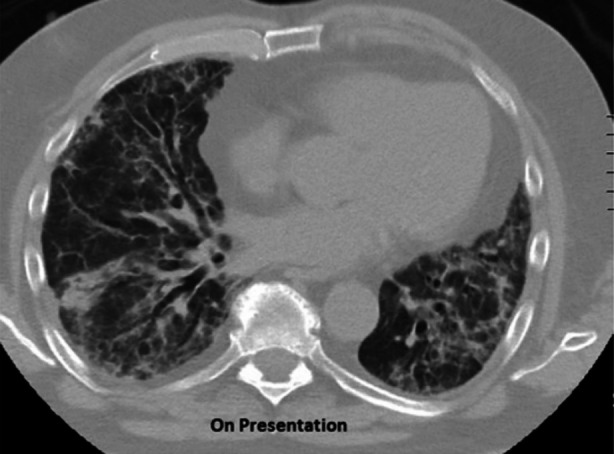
HRCT chest on presentation.
